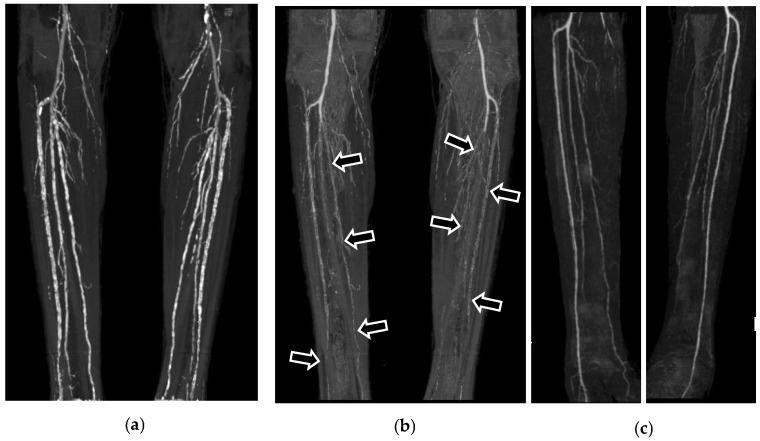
The difference between automatic and semi-automatic manipulation of subtraction CTA with volume position matching. (a) The non-subtracted MIP image shows diffuse arterial wall calcification. (b) The automatically subtracted CTA image, without manual manipulation, shows deletion of the vascular lumen due to misregistration of calcifications (black arrows). (c) The CTA image subtracted using a semi-automatic approach with manual adjustments accurately depicts the vascular lumen.
